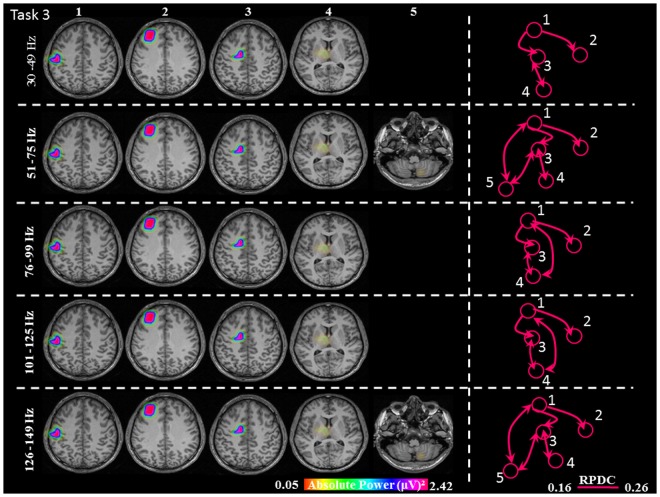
Absolute gamma activity during the continuous Task 3. Estimated sources and direction of information flow in the five frequency bands. The individual maps of power were spatially normalized and interpolated on standard T1-weighted MRI scans with 1 mm spacing in MNI space. The color bar represents the relative power. 1: primary sensorimotor cortex (S1M1); 2: dorsolateral prefrontal cortex (dPFC); 3: supplementary motor area (SMA); 4/5: thalamus (TH)/cerebellum (CER); RPDC, renormalized partial directed coherence.
